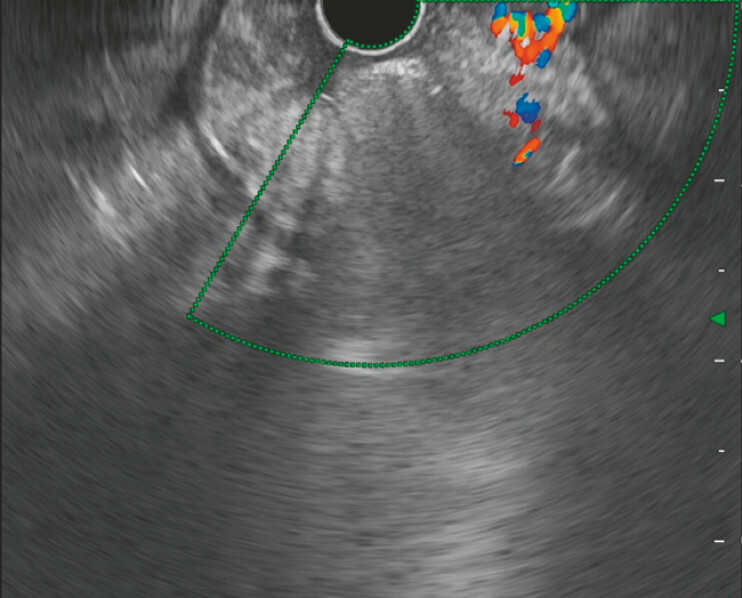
EUS revealed that coils were placed and that the varix flow had disappeared. EUS, endoscopic ultrasound.
